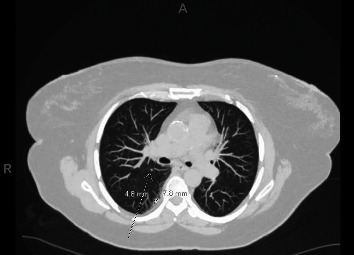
Screening chest computed tomography (CT) scan revealed multiple bilateral pulmonary nodules, measuring up to 7.8 mm.
